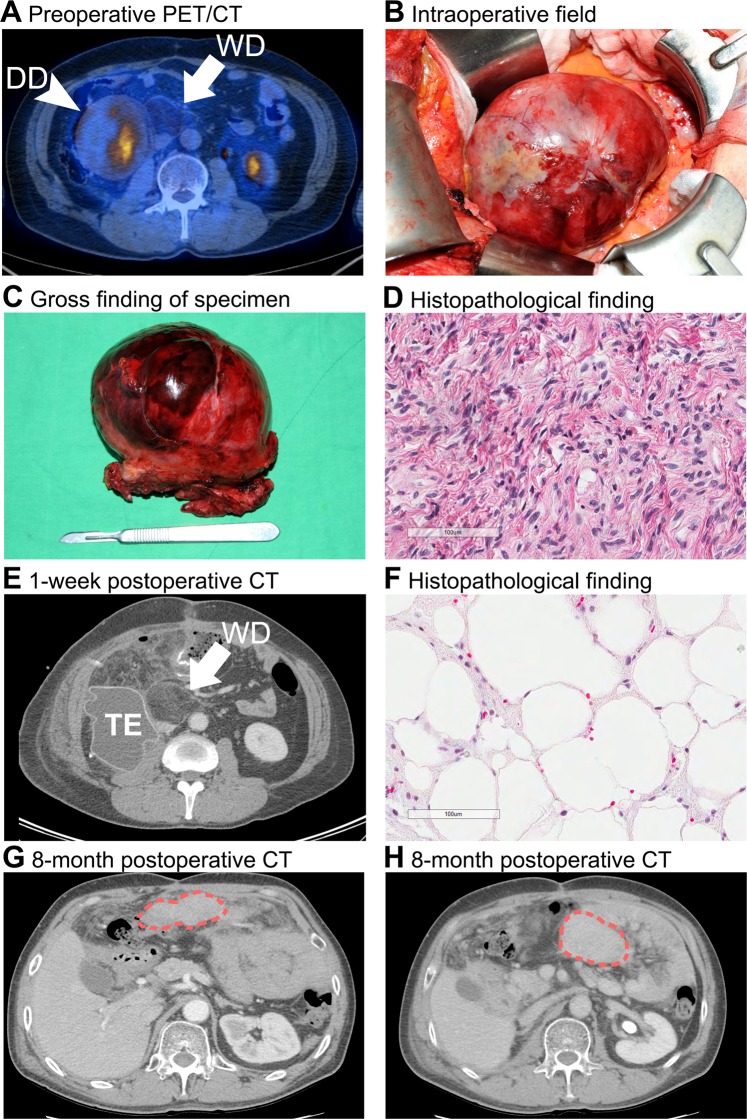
A 56-year-old male patient underwent surgical resection of retroperitoneal LPS. On the preoperative PET/CT scan (A), a hypermetabolic solid mass and a fatty mass with no FDG uptake located on the medial side were detected. A solid LPS was resected (B) and confirmed as DDLPS with FNCLCC grade 2 (C,D). However, the 1-week postoperative CT revealed remnant fatty mass on the medial side (E). After reoperation, the fatty mass was confirmed as WDLPS, FNCLCC grade 1 tumor (F). CT scan performed 8 months after the initial operation revealed peritoneal carcinomatosis with a relatively solid mass (G,H). The patient died 4 months later. Arrowhead indicates DDLPS, arrow indicates WDLPS, red dotted line indicates solid tumor with peritoneal carcinomatosis. DD dedifferentiated liposarcoma, WD well-differentiated liposarcoma, TE tissue expander.
